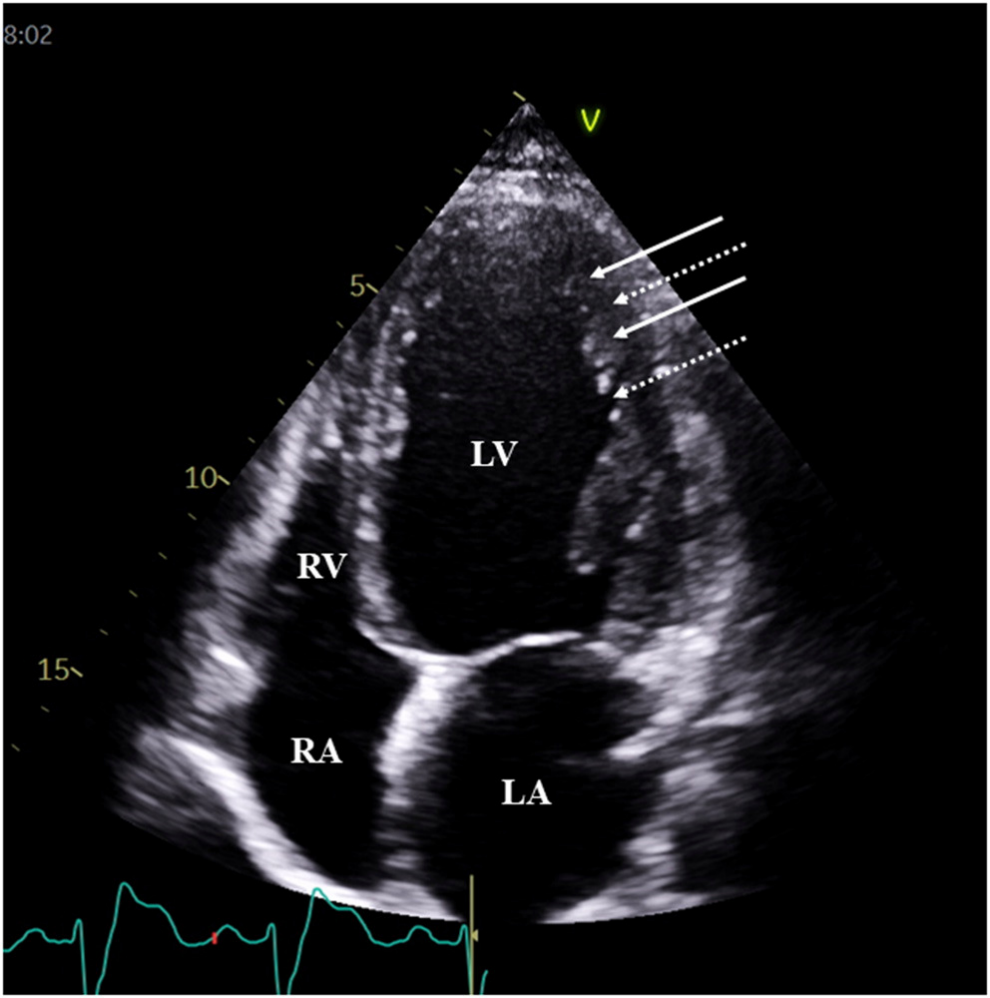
Typical two-dimensional echocardiographic apical four-chamber view demonstrating prominent trabeculations (white arrows) and intertrabecular recesses (dashed arrows) in a patient with typical features of left ventricular non-compaction. White arrows represent trabeculae, while dotted arrows represent sinusoids.
